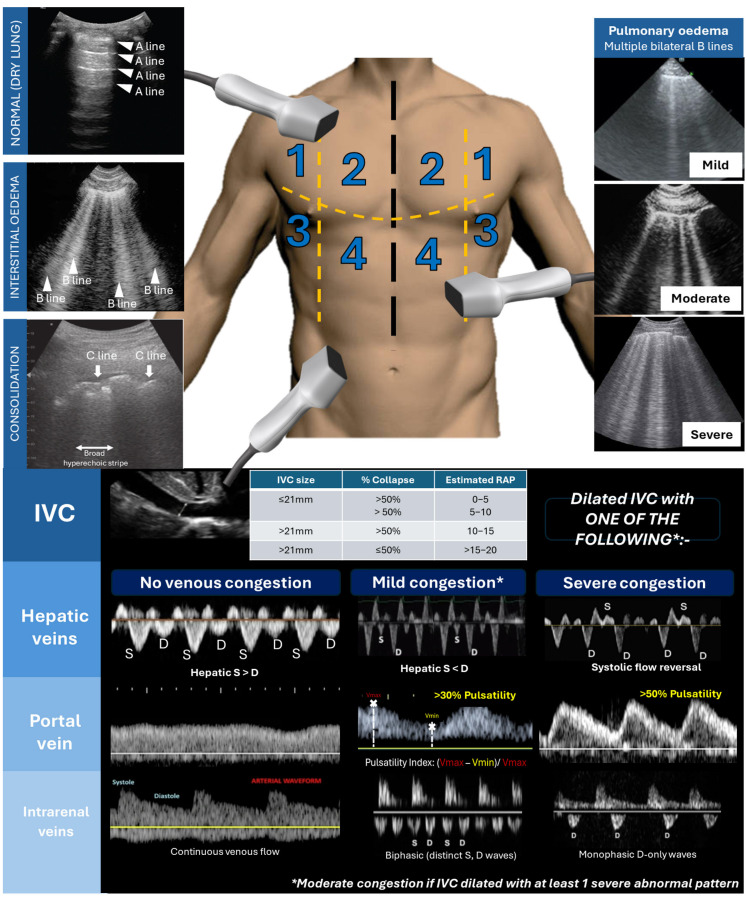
(Top) Thoracic ultrasound to determine the degree of pulmonary oedema (mild: 2–3 B lines; moderate: ≥4 B lines; severe: confluent B lines); C lines: short hyperechoic lines with broad hyperechoic stripe below suggest consolidation. (Bottom) Venous excess ultrasound based on plethoric IVC with abnormal venous waveform in at least one venous system (original figure created by author PT).
